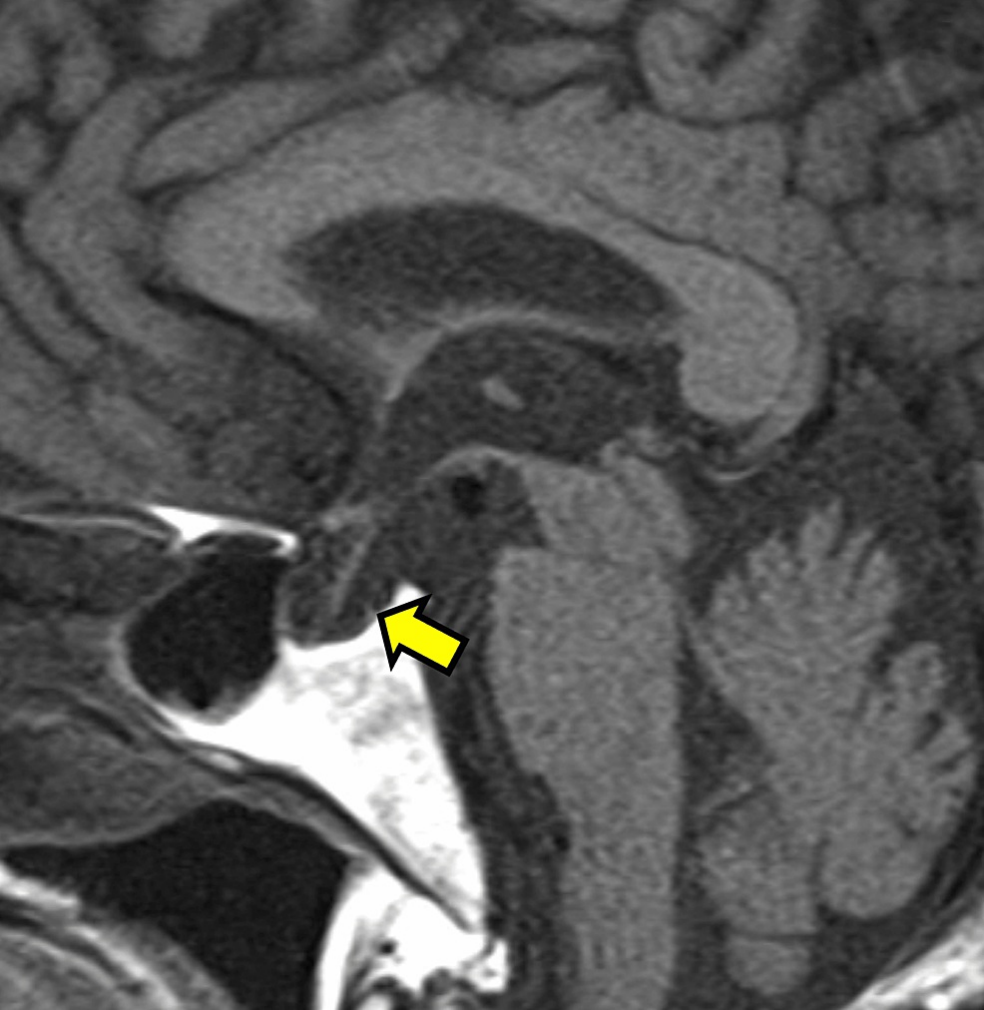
Brain magnetic resonance imaging revealing a thin and flat pituitary gland.
